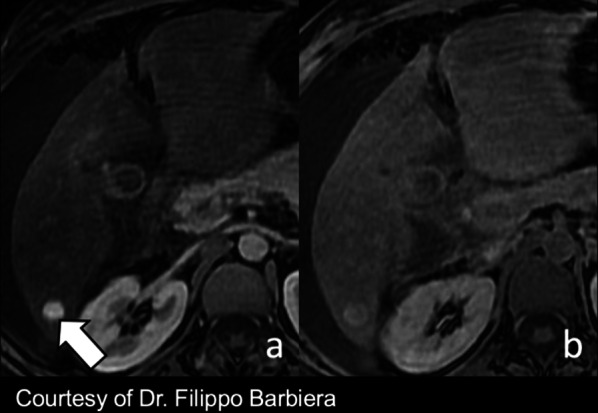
A 38-year old woman with Budd–Chiari syndrome and FNH-like nodule. Gadobenate dimeglumine-enhanced MRI demonstrates a FNH-like nodule that shows (a) arterial phase hyperenhancement (arrow) and (b) hyperintensity in the hepatobiliary phase
